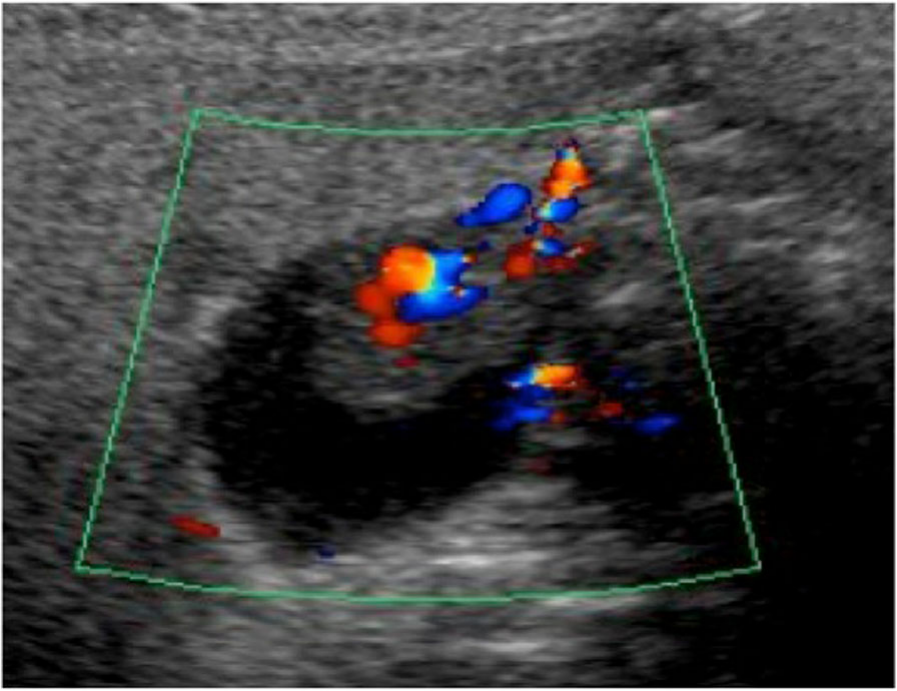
The lesion of the gallbladder was hypervascular by color Doppler US images
